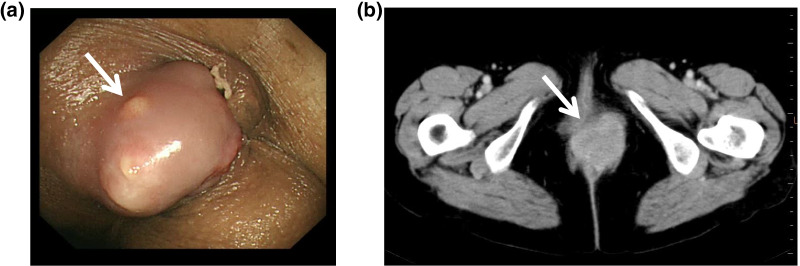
a Endoscopic evaluation revealed an anorectal mass (white arrow). b An enhanced pelvic CT scan showing the mass at the anal canal (white arrow). *CT* computed tomography
